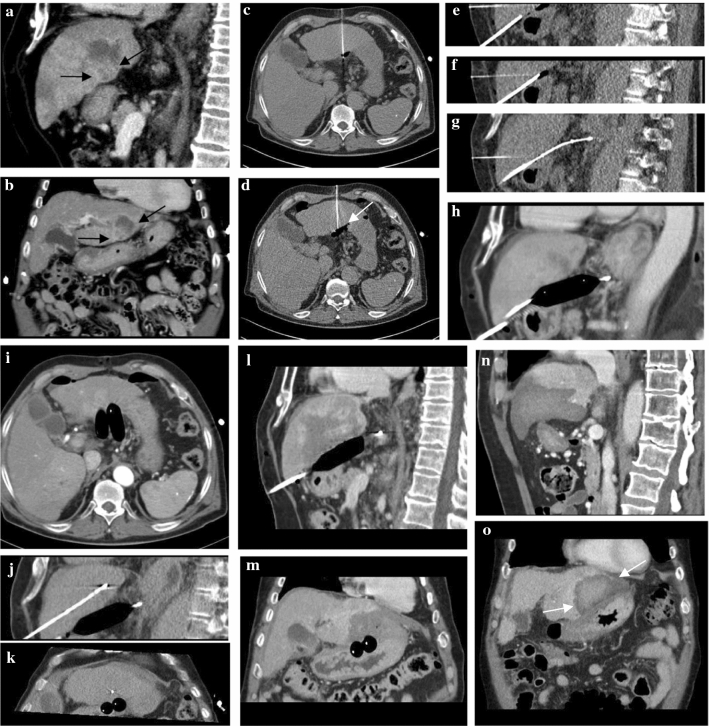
Preinterventional CT scan of a 73y patient with a HCC (black arrows) in S3 adjacent to the stomach (a, b). Insufficient technical success of gas dissection (white arrow, c, d). Positioning of guiding needles in between the liver and the stomach by using blunt trocars (e, f). Advancing angioplasty balloons via 8F sheaths over guiding wires (g, h, i). Placement of the microwave antenna (j, k). CT scan immediately (l, m) and 2 days after thermoablation (n, o) shows the periablational zone covering the entire HCC (white arrows). Complete ablation was confirmed by follow-up. No complication to the stomach occurred
